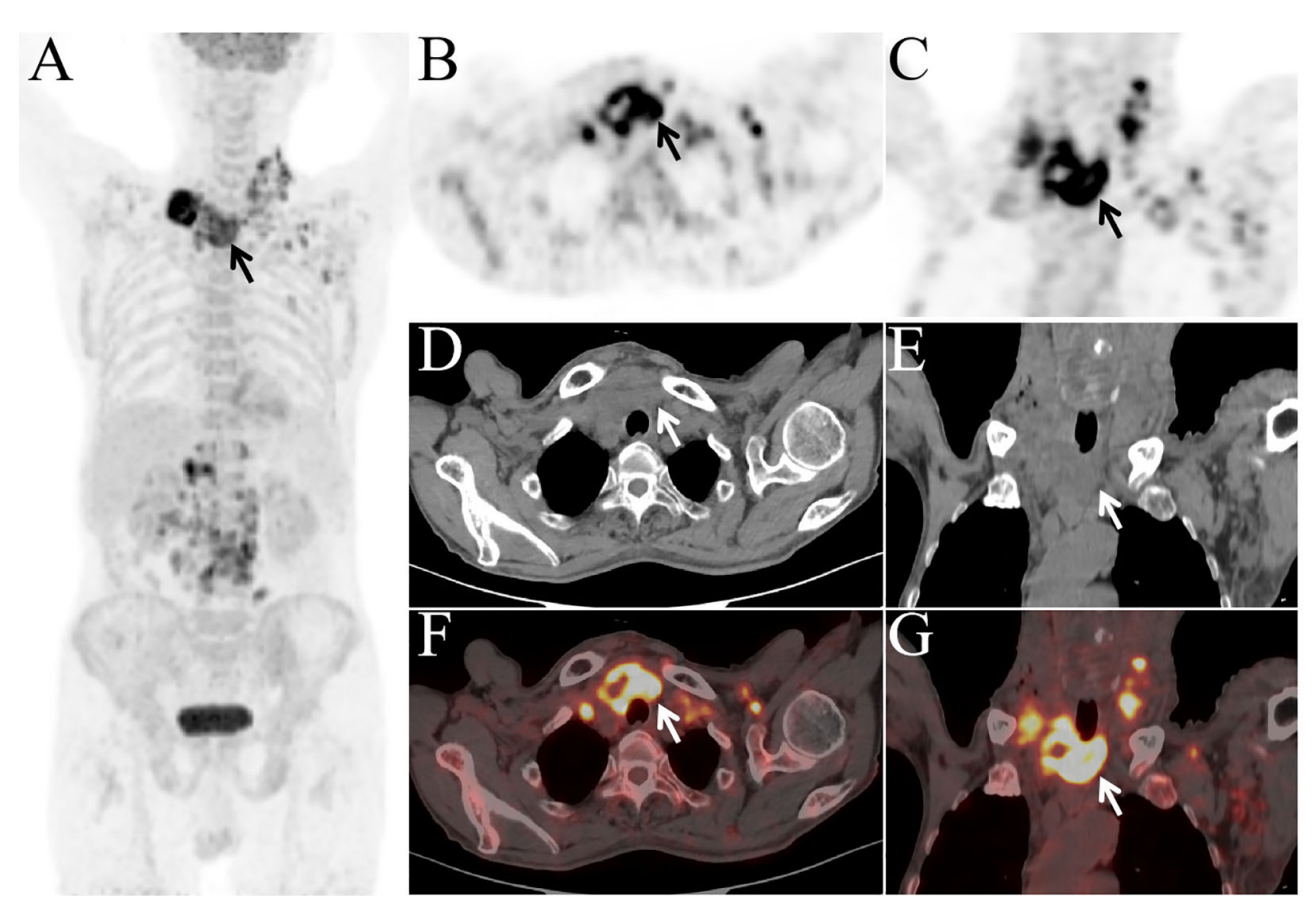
Whole-body imaging (A) revealed multiple areas of increased FDG metabolism in the thyroid region (arrow), neck, and abdomen. The axial and coronal images (B, C), axial and coronal view of PET; (D, E), axial and coronal view of CT; (F, G), axial and coronal view of fusion images) showed a mass in the thyroid with increased FDG metabolism [(B–G), arrow].
